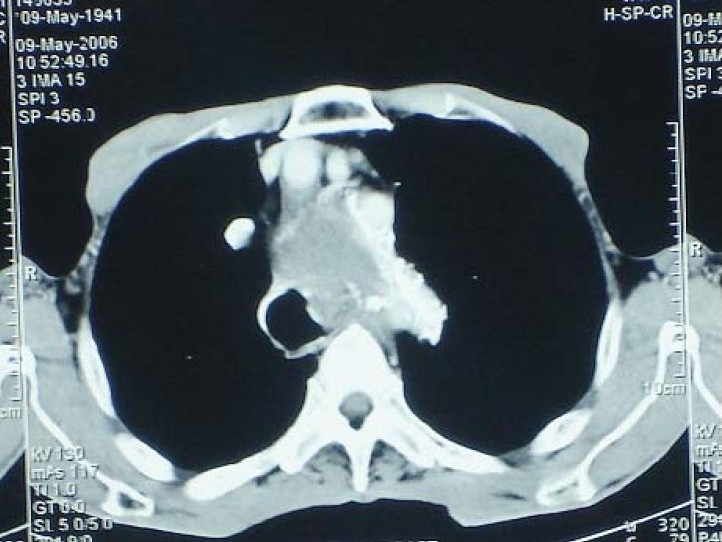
CT Thorax showing the aneurysm bulging into the left wall of Trachea.
